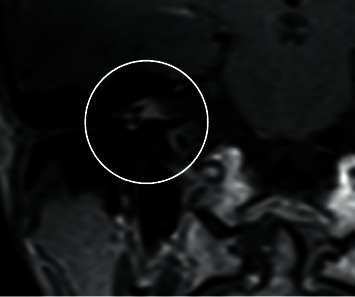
Coronal T1 MRI GAD of cerebellopontine angle 2 years after cochlear implantation of the right ear.
